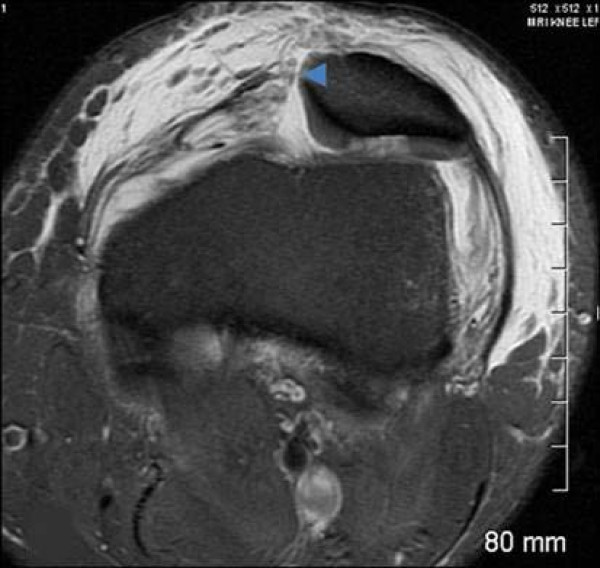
Axial T2 MRI image displays a tear at the patellar attachment (arrowhead).
